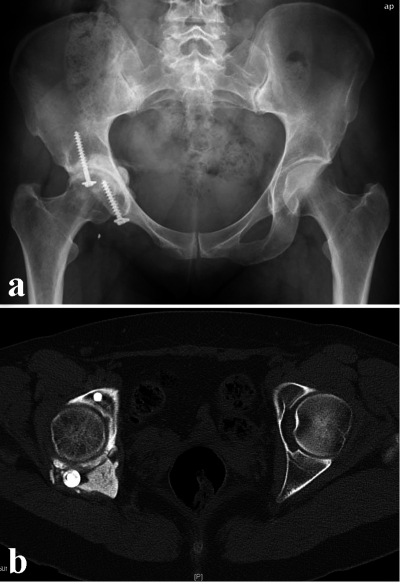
Case 11. a. After intralesional resection of a GCT of the ischium. Additional curettage and bone cement packing of the acetabular extension was performed. The cement was fixed with 2 screws to prevent dislocation. b. CT showing dislocation of the dorsal screw, necessitating surgical removal.
